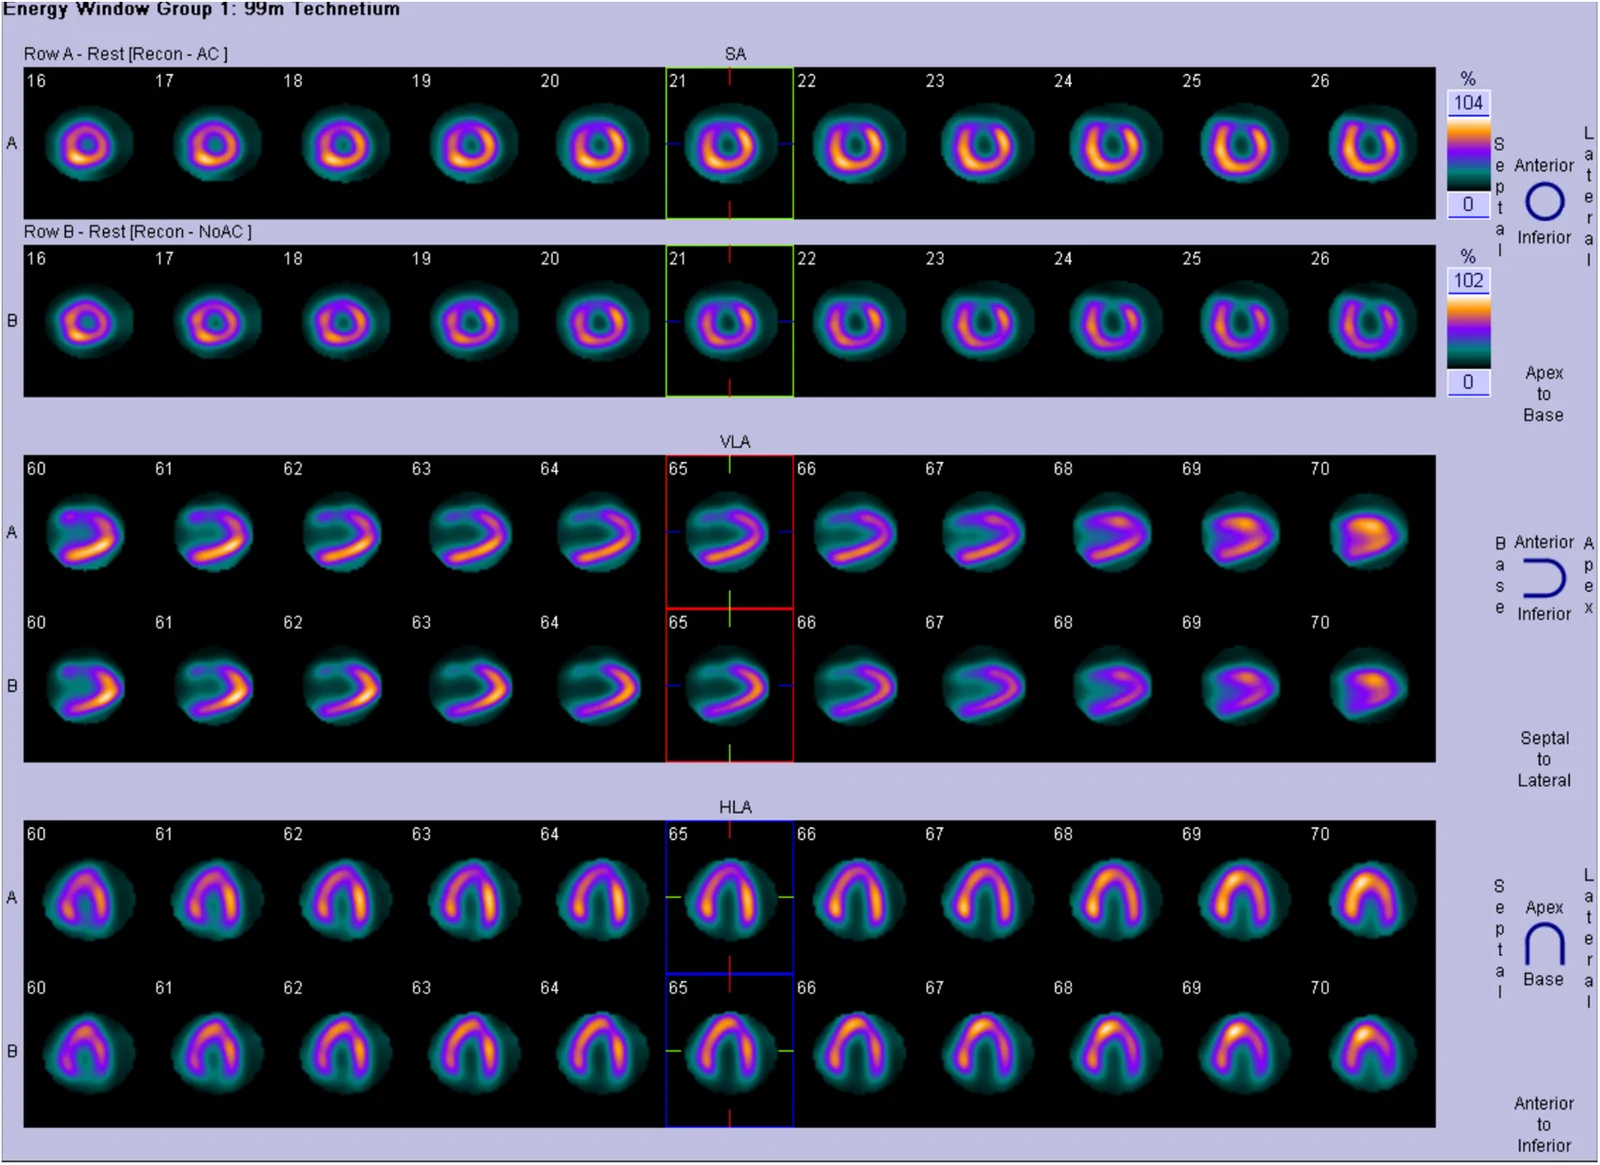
Myocardial perfusion scintigraphy at rest showing significant perfusion defects in the anterior and lateral walls of the left ventricle.
